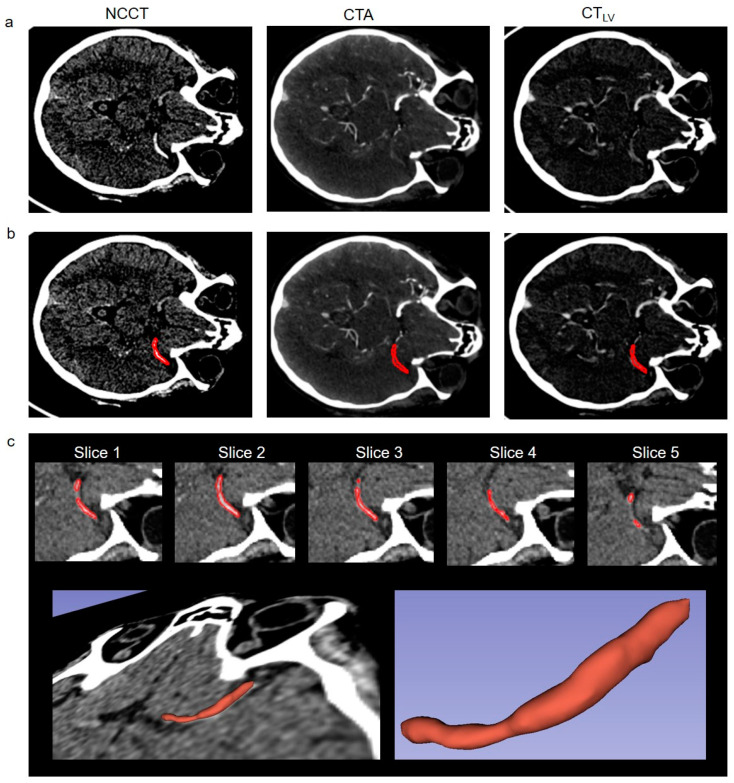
(a) Representative non-contrast CT (NCCT), CT angiography (CTA), and late venous phase CT (CTV) images of our clinical AIS protocol with corresponding segmentation. (b) Corresponding thrombus segmentations for the images in (a) are shown with red. (c) Zoomed-in NCCT images for each thrombus slice position and corresponding segmentation with red. Each 2D transversal slice for the visible clot volume was segmented in the 3D Slicer resulting in full 3D segmentation of the thrombus volume (c). Images show an ICA occlusion in a 70 year old patient with TICI III after intervention.
